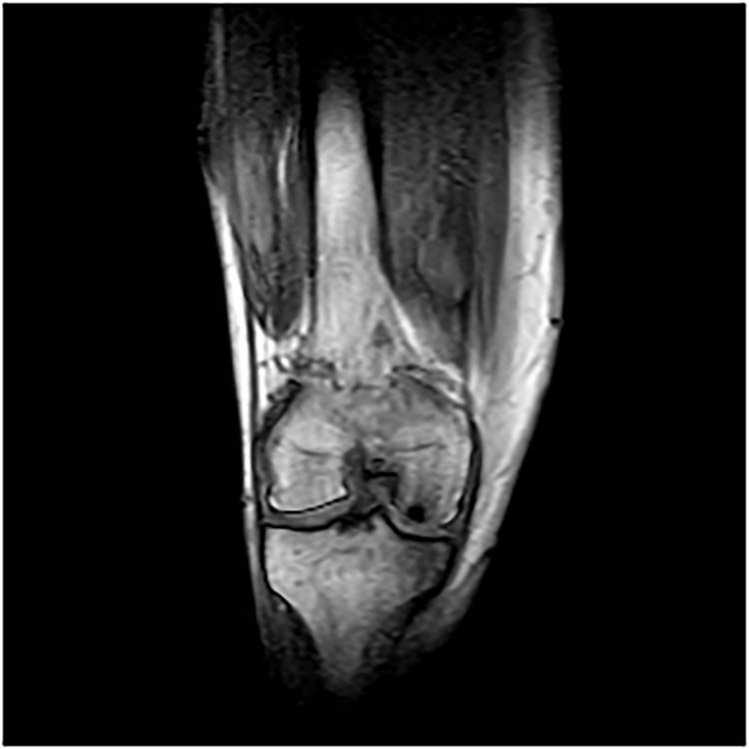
MRI images—osteochondral defect of the knee.
